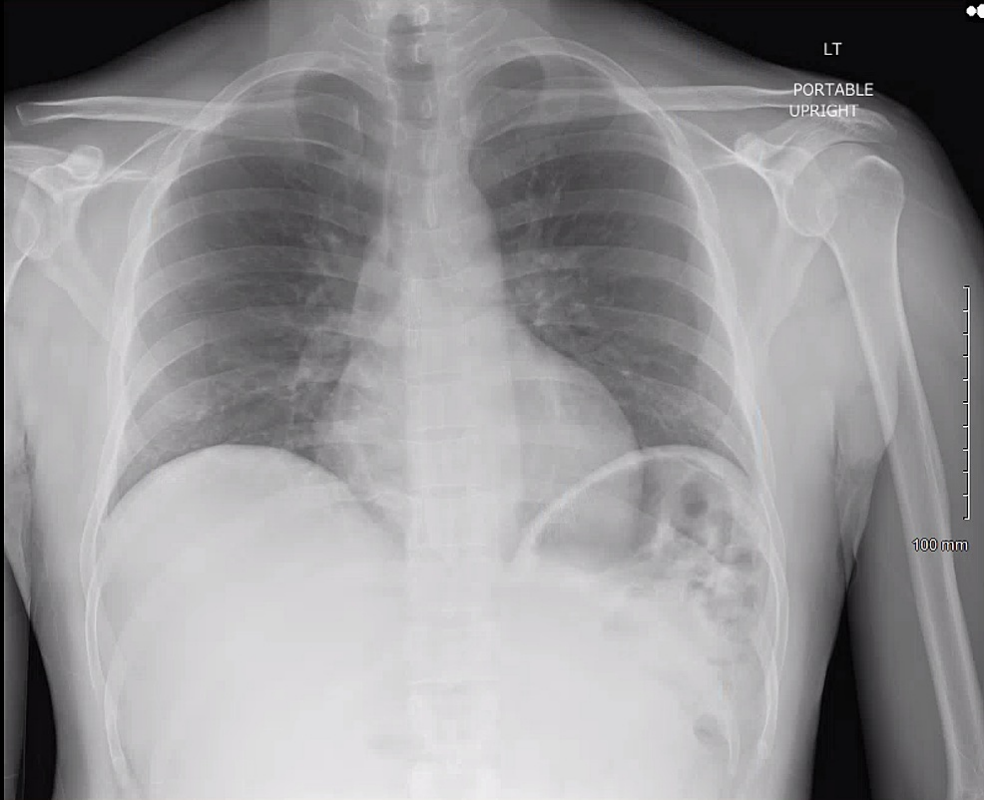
Unremarkable appearing upright chest radiograph.
